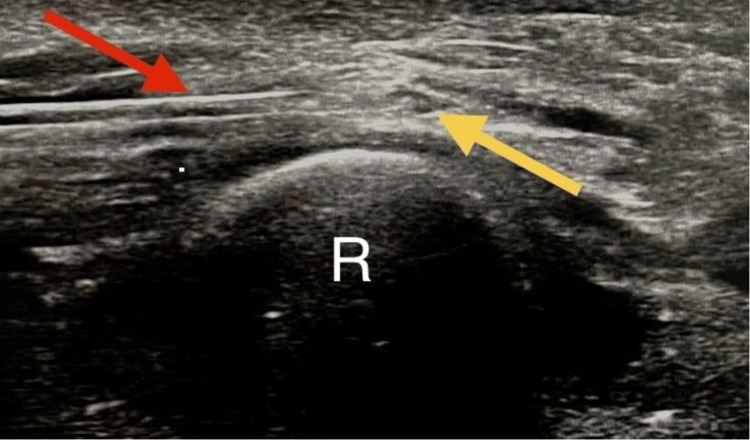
Ultrasonography-guided hydrodissection of the superficial radial nerve. An ultrasound image depicting the superficial radial nerve (yellow arrow) as it courses along the anterolateral border of the radius (R = radius). The image demonstrates the needle (red arrow) positioned for perineural hydrodissection of the nerve.
